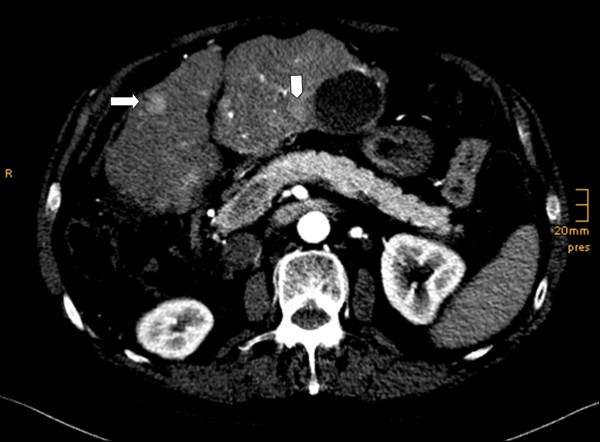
Follow up CT scan six months later. Early arterial examination time scan revealing recurrence of TL3, size 11 mm (arrow) and a new lesion next to a cyst of size 12 mm in liver segment 4b (arrow head).
